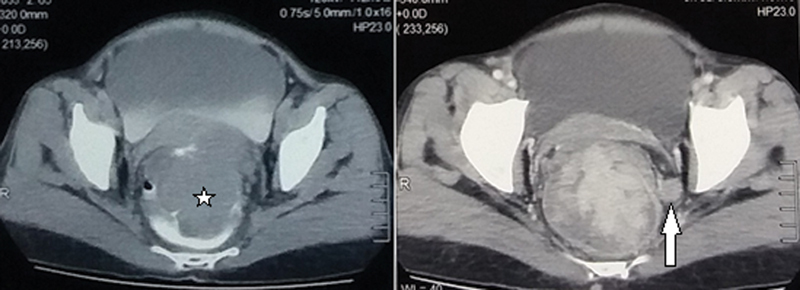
Axial sections of CT pelvis showing large lobulated enhancing intraluminal mass (★) with enlarged perirectal nodes (arrow). CT, computed tomography.
